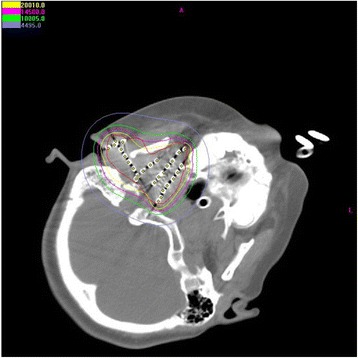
Isodose curve after seed implantation on a CT scan. The *inner red curve* represents the gross tumor volume. The ellipses are isodose lines of 200, 145, 100, and 45 Gy from inside to outside, respectively
